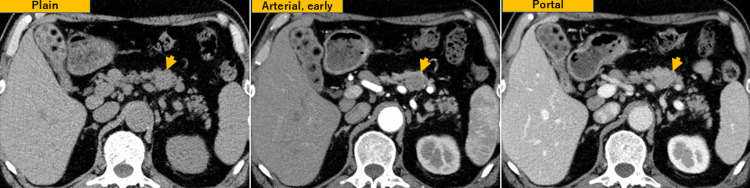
CT images Dynamic CT showed a 20 mm round, expansile mass (arrow head) with a smooth surface in the pancreatic body, without involvement of the main pancreatic duct. The mass was mildly enhanced in the early phase and showed gradual and marked enhancement in the delayed phase.
